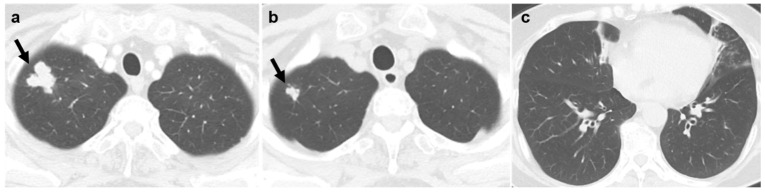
A female patient with nontuberculous mycobacterial infection. (a) Chest CT demonstrates a 2.8 cm lobulated irregular nodule (arrow) in the right upper lobe, confirmed as squamous cell carcinoma by percutaneous core needle biopsy. (b) On CT scan obtained 18 months earlier, the nodule measured 1.2 cm (arrow). (c) Mild bronchiectasis and nodular infiltration are observed in the right middle lobe and the lingular segment of the left upper lobe.
